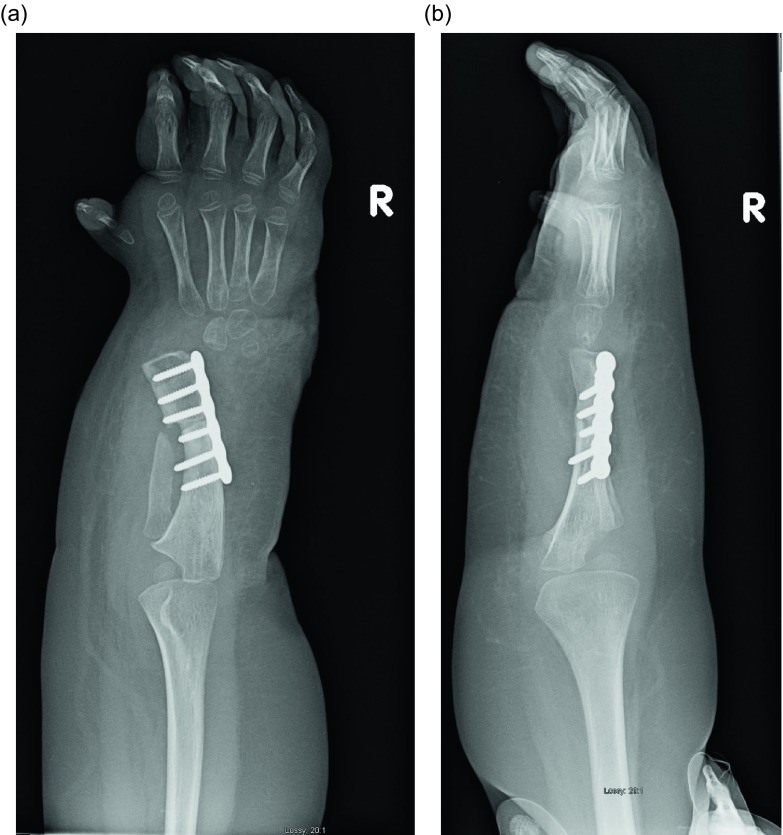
AP and lateral radiographs after removal of the wires. Note that the ulnar head remains at station.
